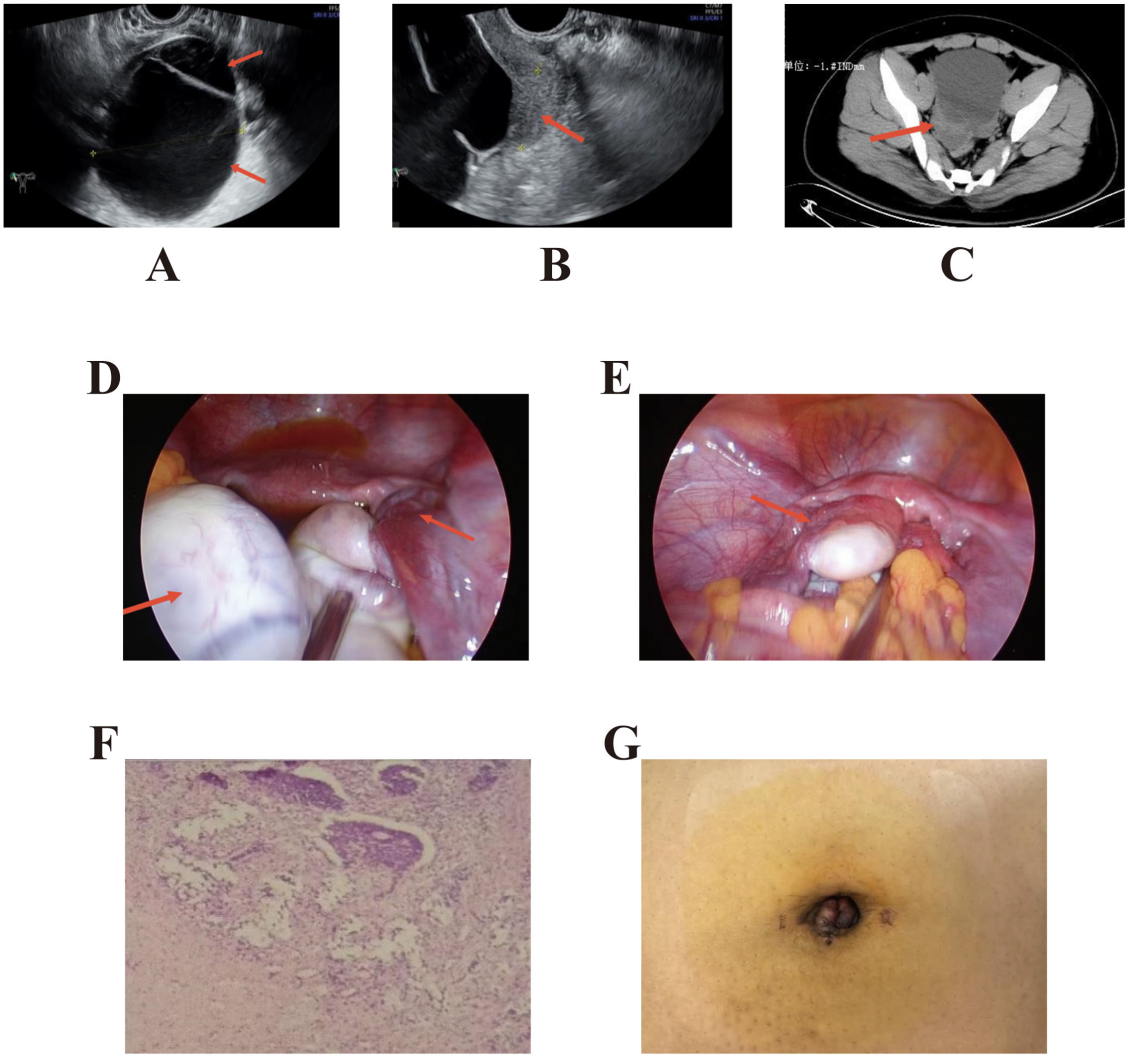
(A) Ultrasound on admission day showing a large cystic mass in the right adnexal region, approximately 12.0 × 9.4 × 9.1 cm in size, with clear margins, anechoic interior, and visible septal reflections. (B) Dynamic ultrasound observation revealing a “whirlpool sign.” A mixed-echo mass, measuring approximately 4.8 × 4.7 cm, was observed between the cyst and the uterine body. Real-time imaging demonstrated whirl-like changes, indicating pedicle torsion. (C) Whole-abdominal CT from another hospital showing a pelvic cystic mass with pelvic effusion and fatty liver changes. (D) Single-port laparoscopic exploration revealing partial cystic enlargement of the right ovary (approximately 12 × 10 cm), with an additional 2 × 1 cm protruding cyst. Right adnexal torsion was observed at 540°, with minimal pelvic fluid. (E) Intraoperative view showing the right ovary reduced into the pelvis following extracorporeal manipulation, complete cyst wall dissection, and suture reconstruction after ovarian plication. (F) Postoperative pathology showing a right ovarian follicular cyst with mild granulosa cell hyperplasia. (G) Postoperative umbilical incision appearance after cosmetic suturing using the “anchor fixation technique”.
